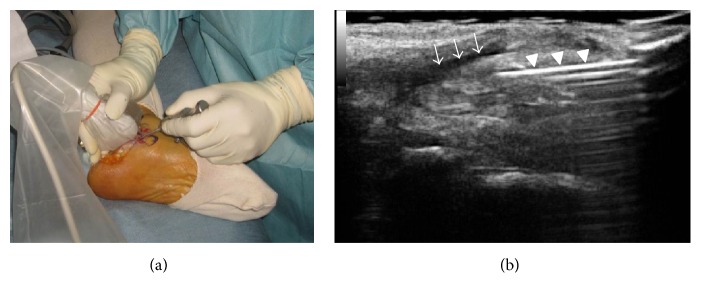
Sonographic findings. Macroscopic (a) and sonographic (b) findings. Arrows show the irrigation fluid and arrowheads show the needle.
